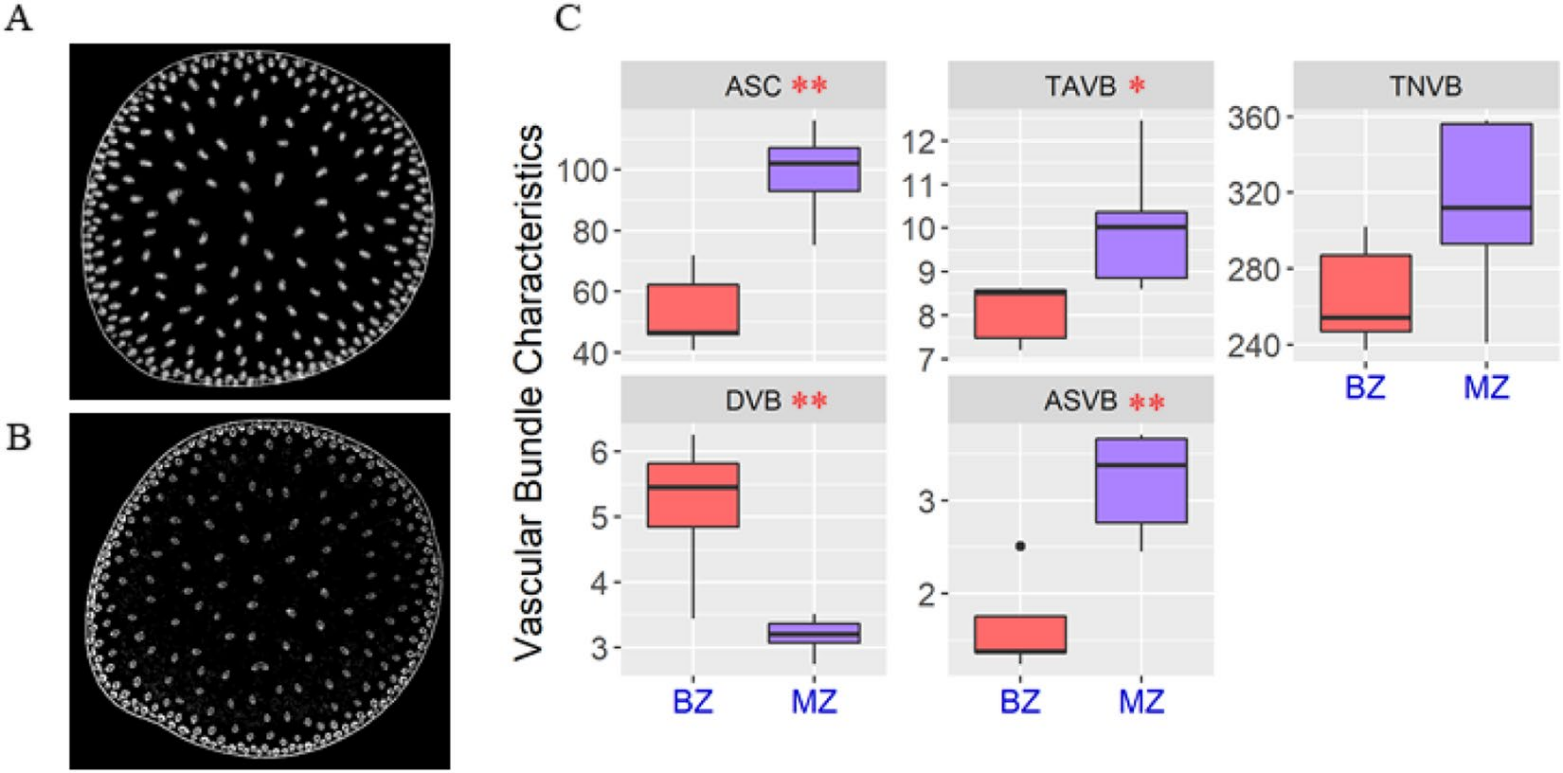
X-ray microcomputed tomography images and vascular bundle traits of the BZ and MZ tissues. (A) X-ray microcomputed tomography image for the BZ. (B) X-ray microcomputed tomography image for the MZ. (C) The distributions and differences of five traits related to vascular bundles for the BZ and MZ, respectively. TNVB: total number of vascular bundles; ASC: area of the stalk cross section; DVB: density of vascular bundles; ASVB: area of a single vascular bundle; TAVB: total area of vascular bundles. Five samples were used for the BZ and MZ, respectively. * and  indicate the significance at the 0.05 and 0.01 levels, respectively.
